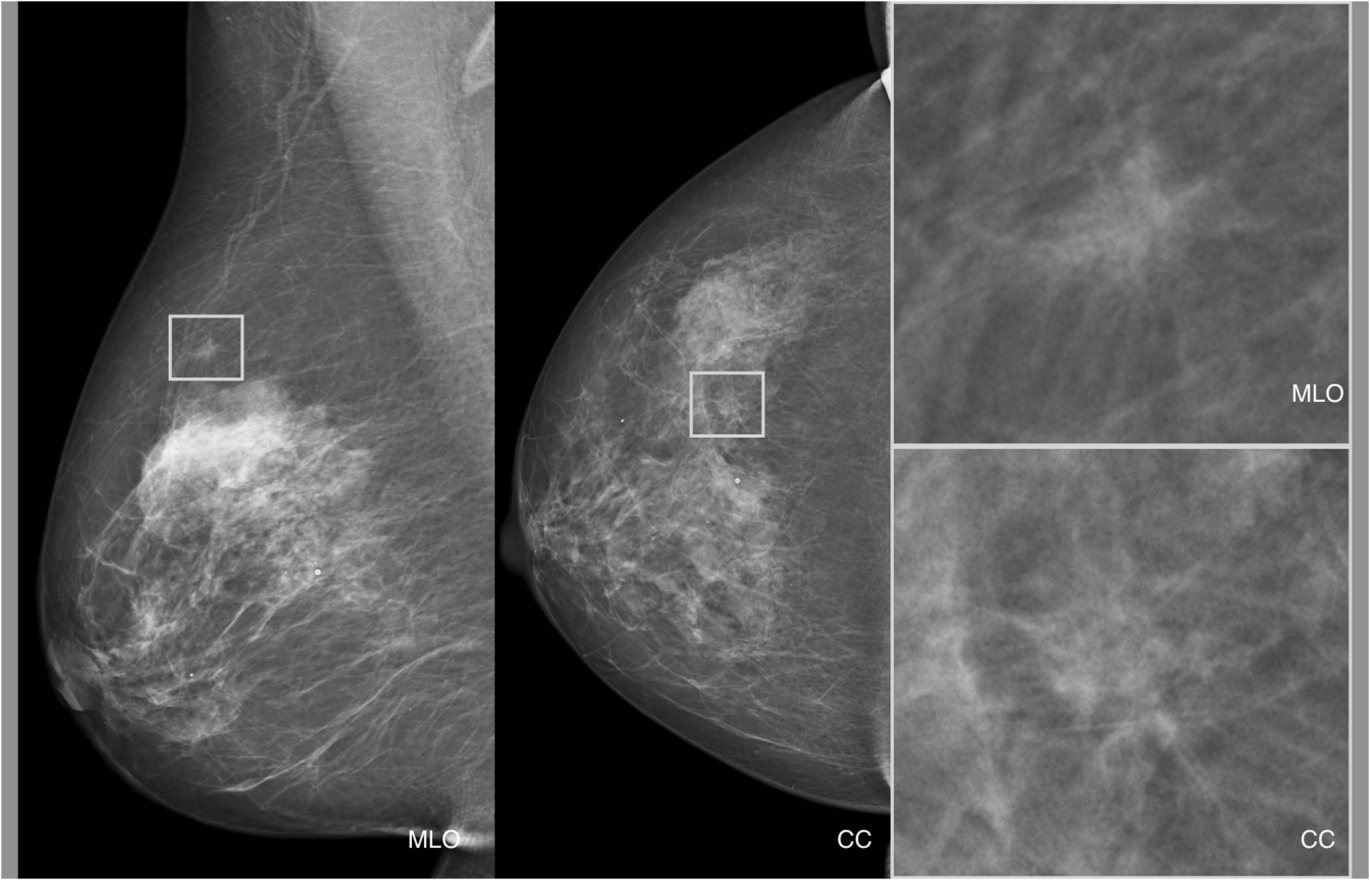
A cancer missed by the AI system. A 7-mm-large invasive tubular cancer (grade 1) with the radiographic appearance of a spiculated mass that was categorised with an AI risk score of 3. MLO, mediolateral oblique view; CC, craniocaudal view
